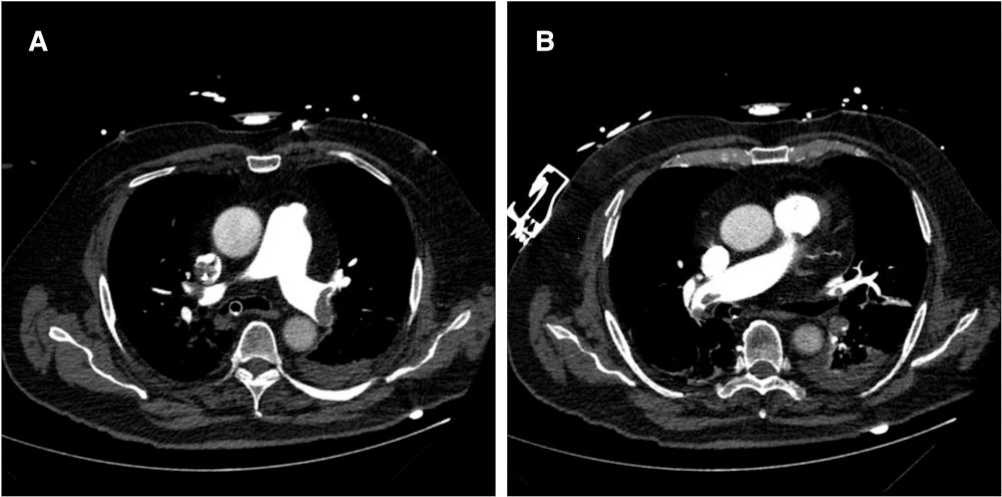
(*A*) Axial slice of computed tomography pulmonary angiography (CTPA) visualizing central thrombotic sub-total occlusion of the right and left pulmonary artery (PA). (*B*) Axial slice of CTPA visualizing central sub-total thrombotic occlusion of the right PA.
